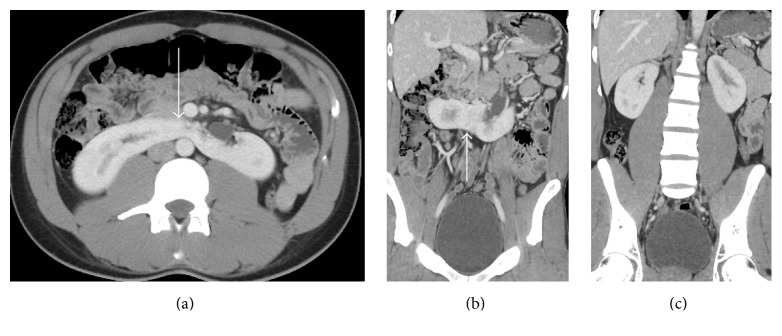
27-year-old male with horseshoe kidney. Axial (a) and coronal (b) contrast-enhanced CT images show the inferior aspect of a horseshoe kidney in the lower abdomen. Note the enhancing isthmus of renal parenchyma crossing the midline (arrow). A coronal contrast-enhanced CT image (c) obtained more posteriorly shows the upper poles of the horseshoe kidney in the renal fossae.
